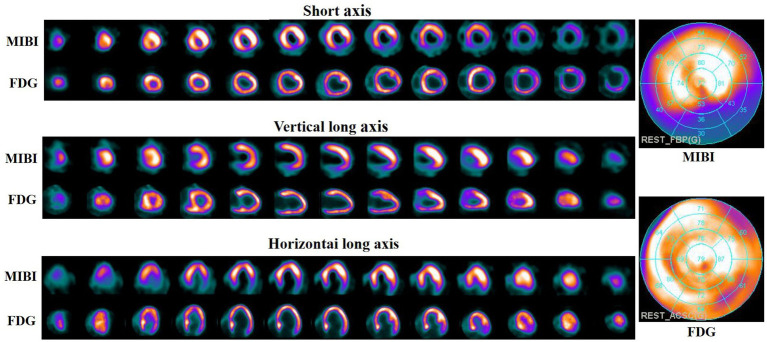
Rest myocardial perfusion ^99m^Tc-MIBI SPECT images demonstrating mismatch with ^18^F-FDG PET images in patient with CHD. SPECT shows moderate hypoperfusion in inferior and inferolateral walls, whereas PET shows normal ^18^F-FDG uptake, consistent with hibernating myocardium.
